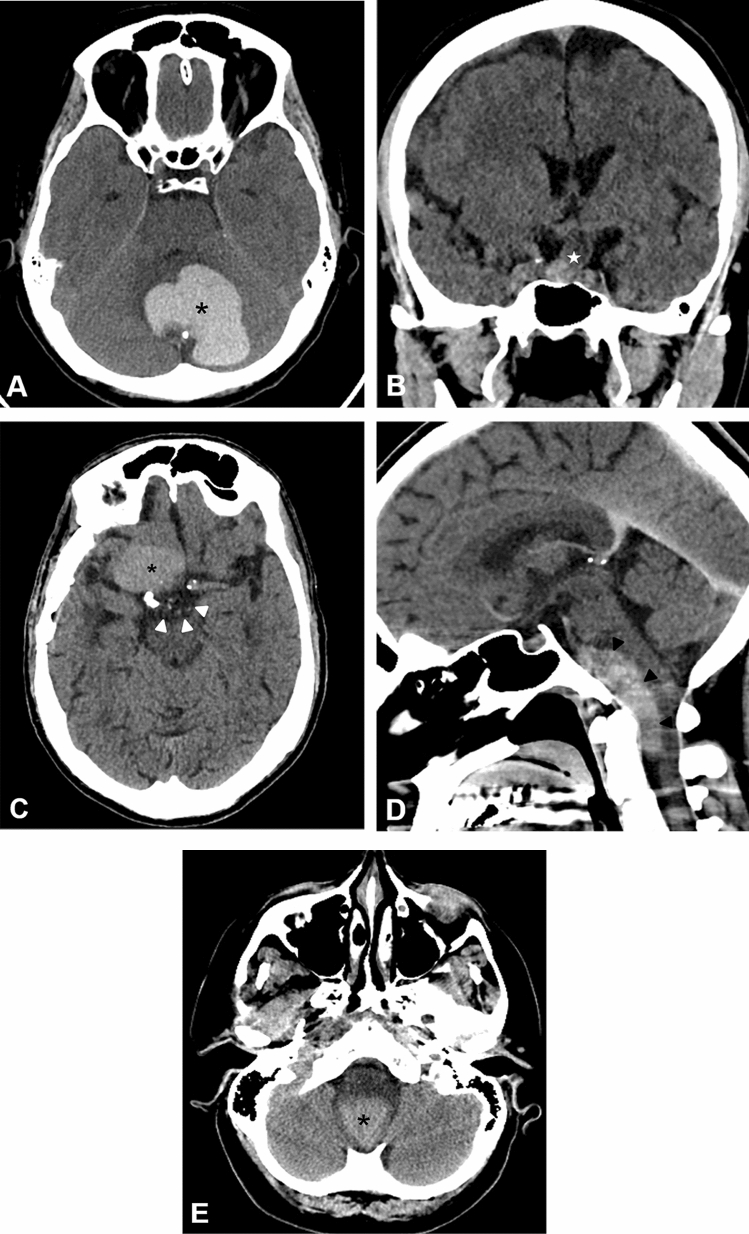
Plain CT scan images of the brain of the 5 patients with white epidermoid cyst. The lesions exhibit variable spontaneous hyperdensity. Patient 1 (A) has a lesion in the posterior fossa in the retrocerebellar region (black asterisk) with a small focus of peripheral calcification. Patient 2 (B) presents with a sellar/suprasellar hyperdense lesions (white star). Patient 3 (C) has an epidermoid cyst with 2 components: classic epidermoid (white arrows) in the suprasellar region and white epidermoid (black asterisk) in the right middle cranial fossa, which developed after partial resection. Patient 4 (D) presents with a lesion located in the posterior fossa anteriorly, extending to the craniocervical junction (black arrowheads). Patient 5 (E) has a foramen magnum epidermoid cyst with extension to the spinal canal (black asterisk).
